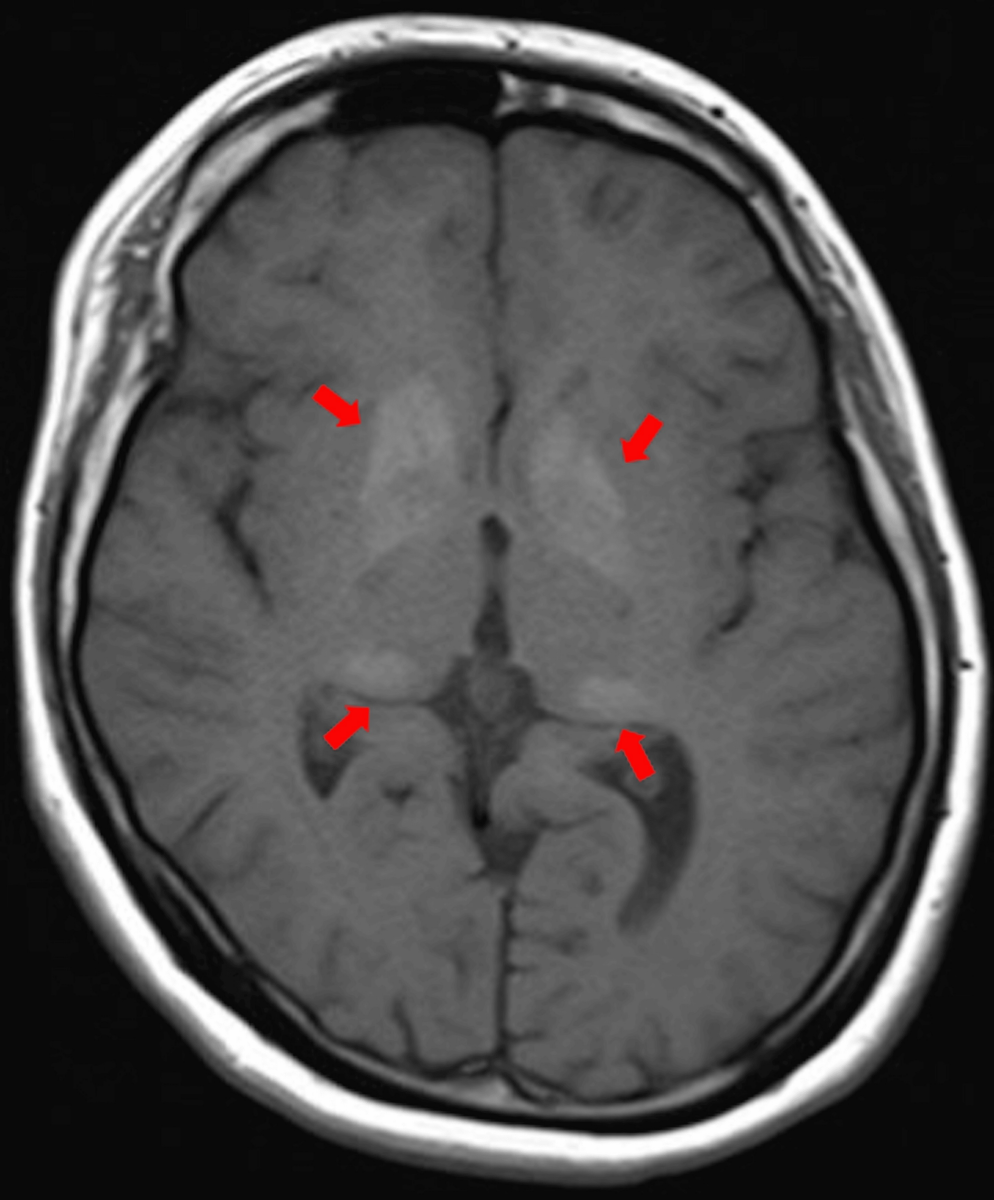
Brain MRI (T1-weighted imaging) at age 21 T1-weighted brain MRI shows faint hyperintensities in the basal ganglia (red arrows), which were not present on childhood imaging. These MRI changes correspond to the calcifications demonstrated on non-contrast head CT at the same age.
